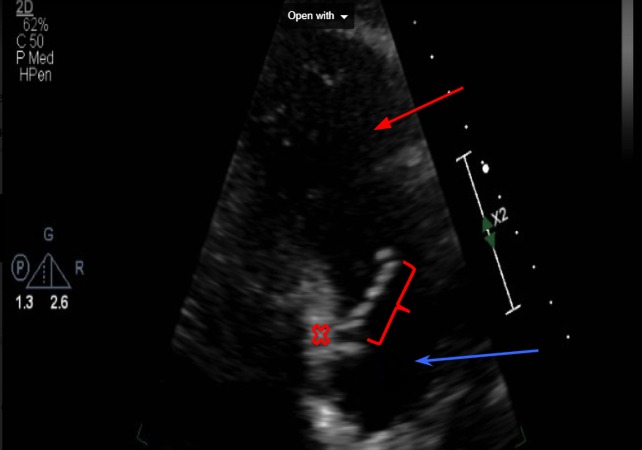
Right-sided 2D echocardiogram showing the vegetation adhered to the right interatrial septum Red bar = vegetation, red x = interatrial septum, blue arrow = right atrium, red arrow = right ventricle 2D: two-dimensional
